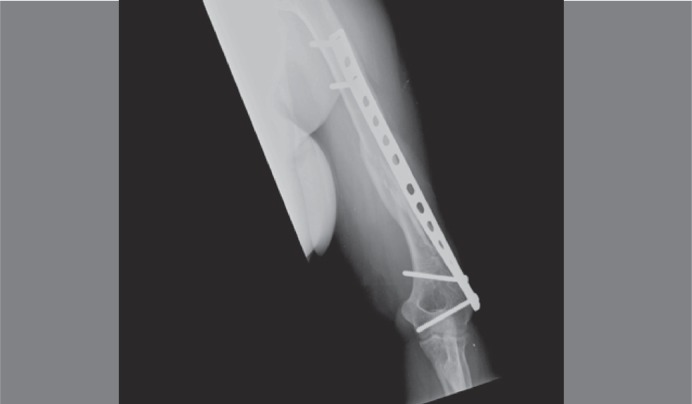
Postoperative X-Ray (AP) of patient treated with MIPO technique and direct visualization of the radial nerve.
